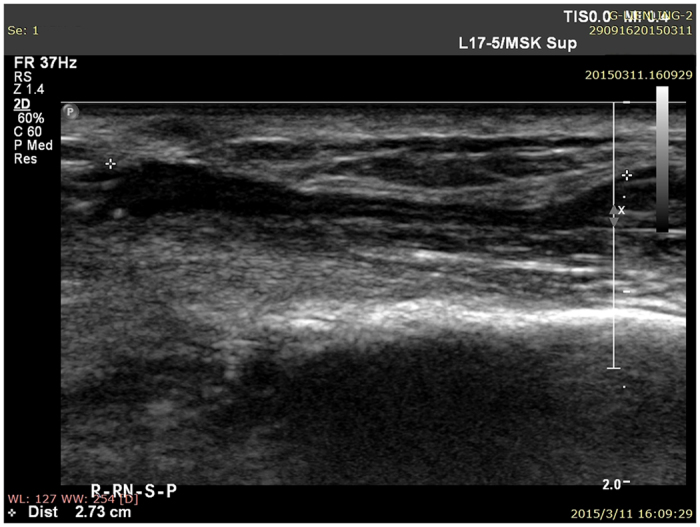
Regenerated superficial radial nerve in high-frequency ultrasound at three postoperative months.
